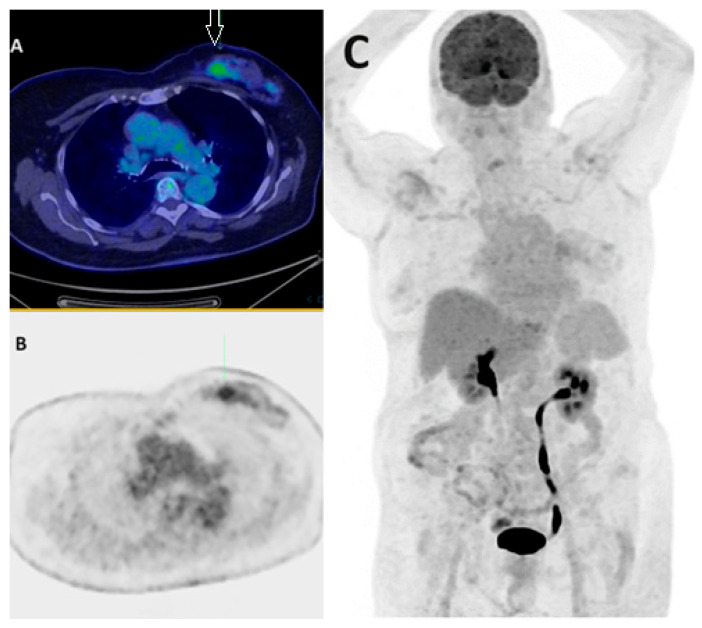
Lower FDG avidity in certain BC subtypes. Fused axial PET/CT (A), axial PET only (B), and MIP PET/CT (C) demonstrate low-grade FDG uptake in biopsy-proven left breast lobular carcinoma (arrow) in a 55-year-old patient. The lower SUVmax of invasive lobular carcinoma (ILC) primary tumors compared to invasive ductal carcinomas (IDCs) is attributed to factors such as lower tumoral cell density and reduced proliferation rates.
